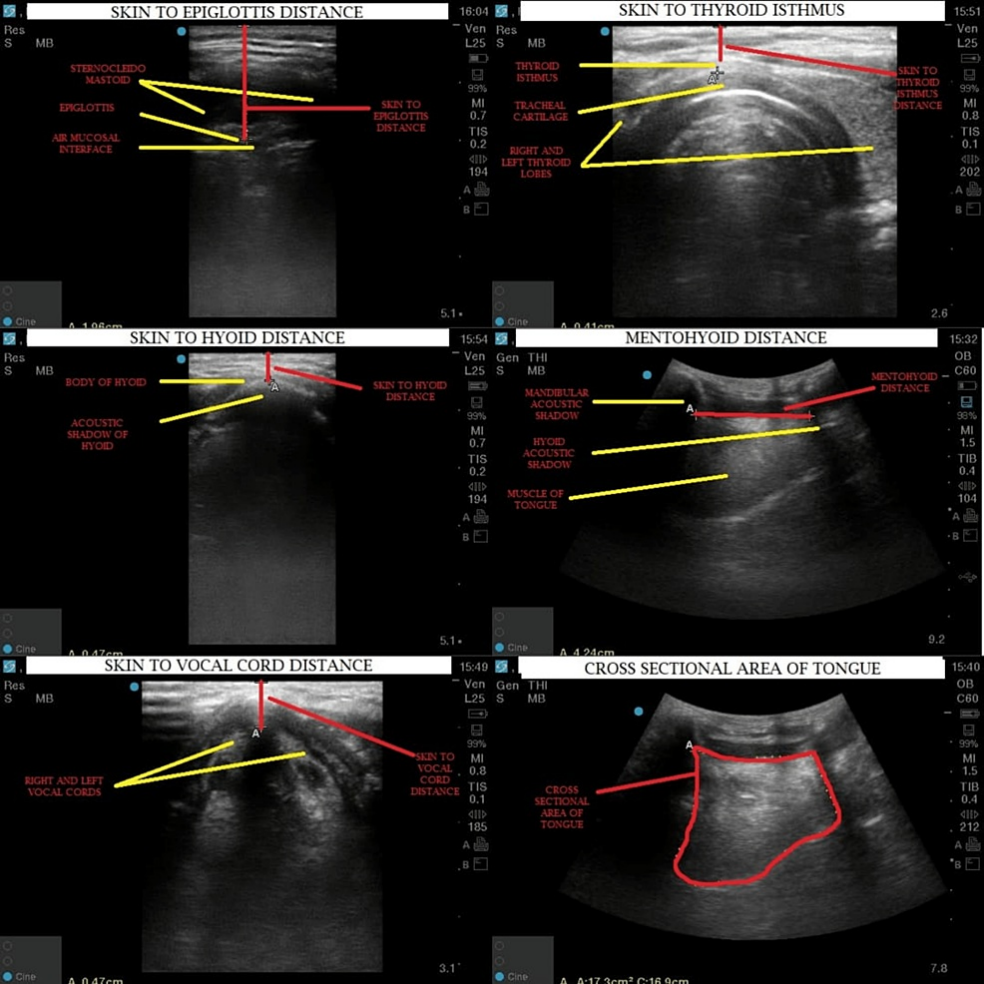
Various ultrasonographic parameters.
